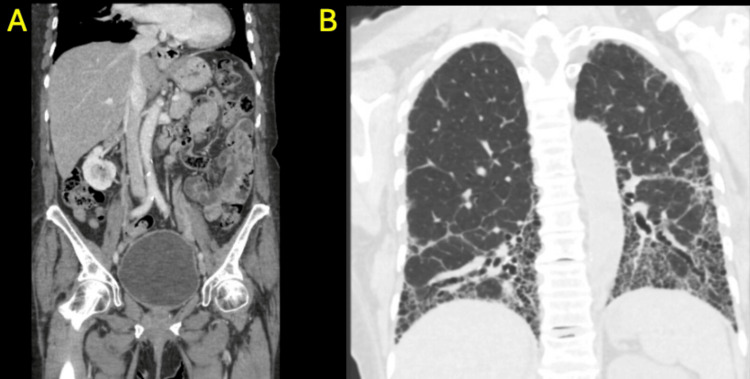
CT of the abdomen/pelvis with contrast and CT of the chest without contrast CT of the abdomen/pelvis with contrast (A) did not demonstrate malignancy but showed multilevel thoracolumbar spondylosis and diffuse hepatic steatosis. CT of the chest without contrast (B) showed extensive subpleural fibrosis of lower lobes left-greater-than-right with extensive honeycombing and bronchiectatic changes in lower lobes, right middle lobe, and left upper lobe. CT: computed tomography
